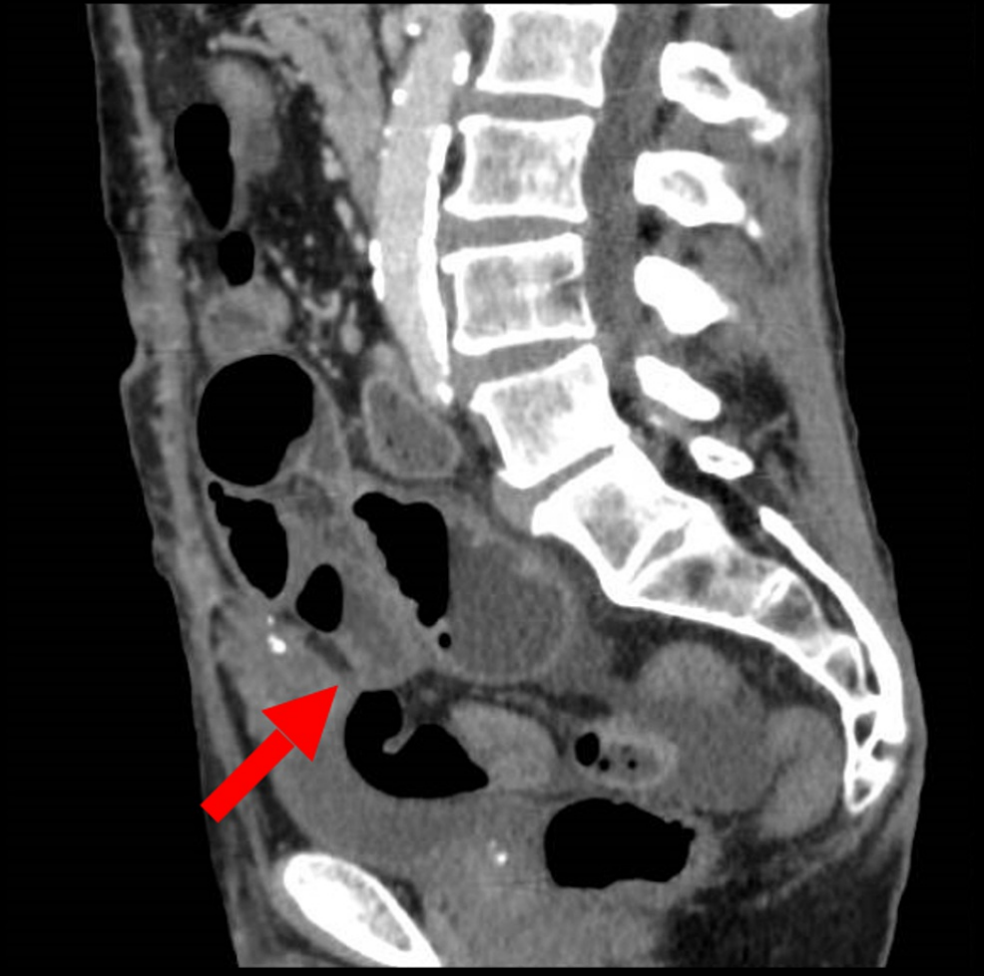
CT scan - sagittal section Arrow indicates the presence of urachal remnant CT: computed tomography
